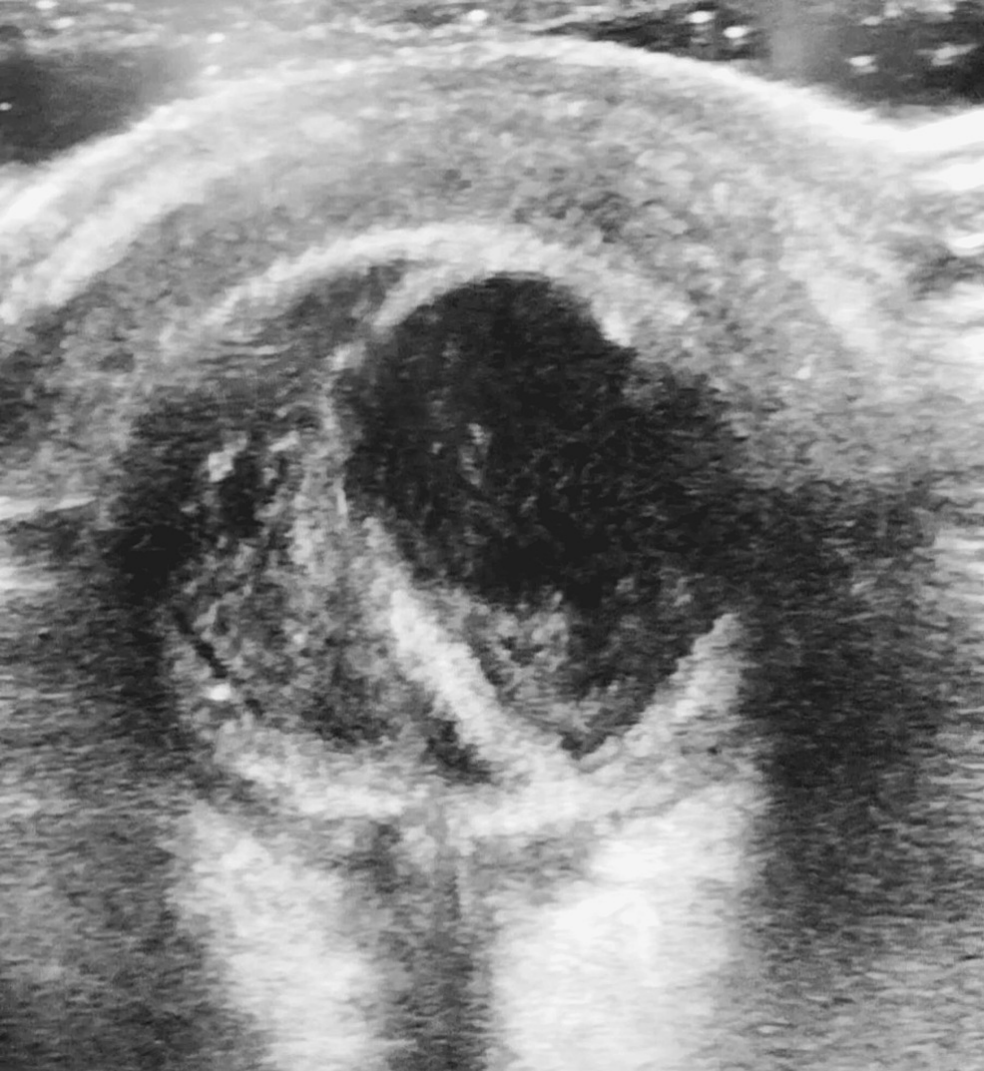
Ultrasound B-scan findings The image showed scleral thickening with a detached retina and vitreous echoes suggestive of panophthalmitis
